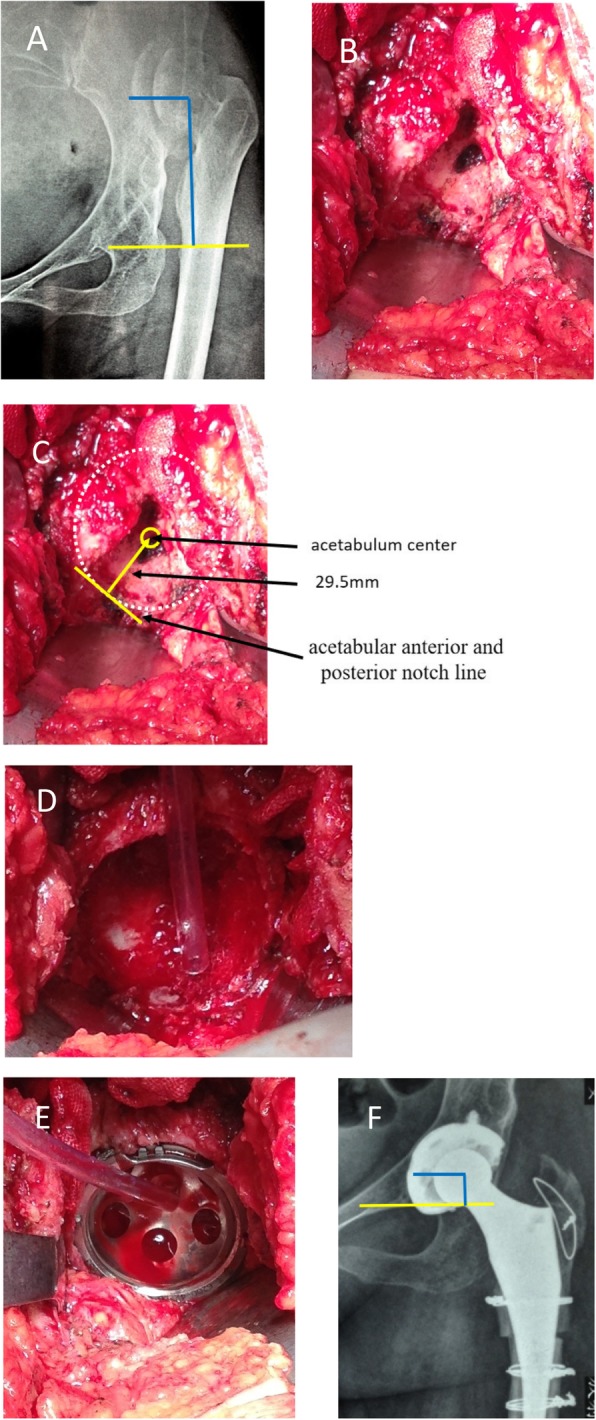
Radiographs of a 52-year-old female patient with end-stage osteoarthritis secondary to Crowe type IV DDH. a Preoperative X-ray. b Intraoperative image showed the triangular-shaped acetabulum and shallow-shaped Harris fossa. c Locating the acetabulum center. d Reaming the acetabulum. e Installing the acetabular cup. f Postoperative X-ray and rotation center restoration
